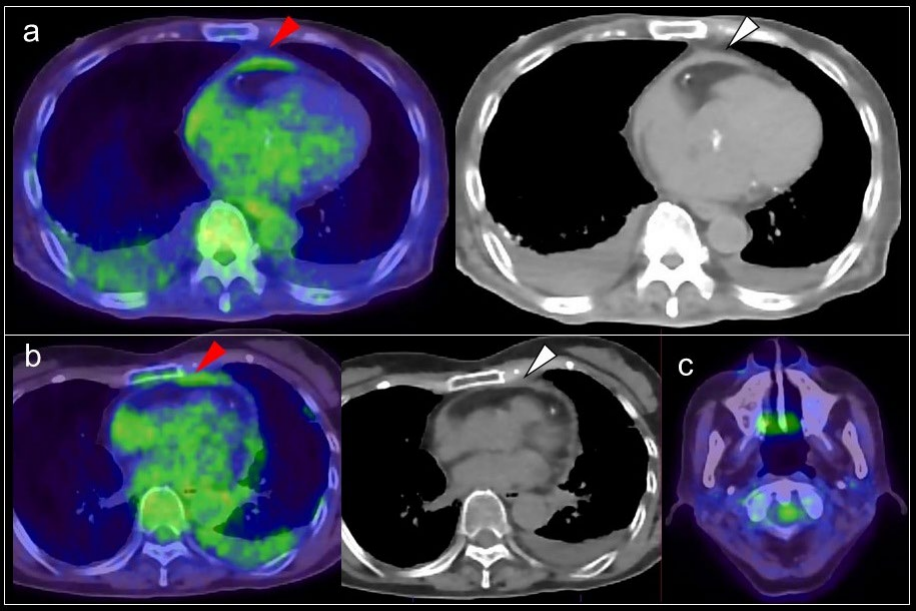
Pericardial lesion associated with systemic disease. a FDG uptake could be confirmed in the thickened pericardium, suggesting pericardium associated with SLE. b Moderate FDG uptake was seen in the part of thickened pericardium. It was acute pericarditis occurred in the patient was Sjögren syndrome showing the atrophic change in both parotid grands (c)
